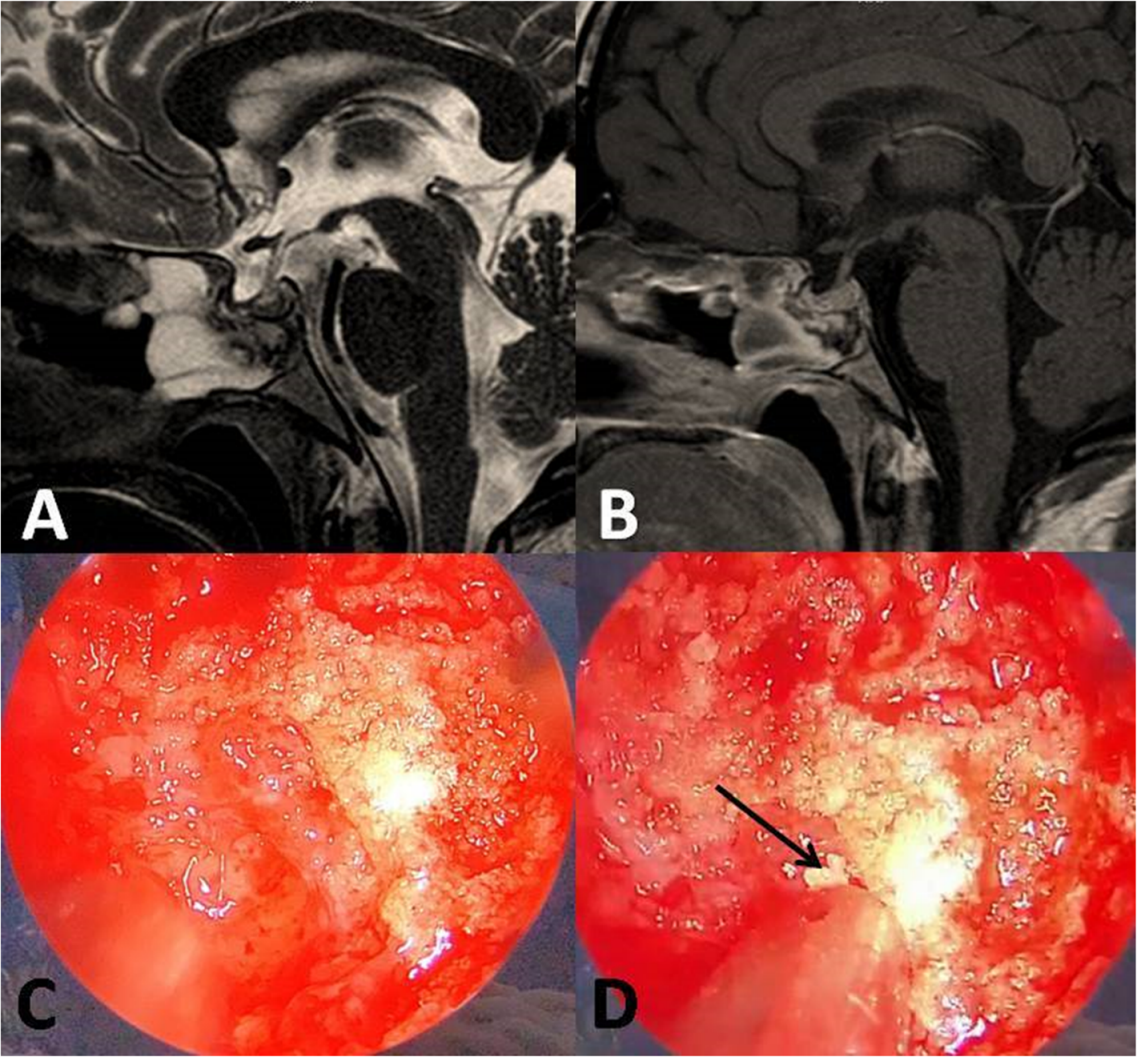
a) T2-weigthed and b) post-contrast sagittal brain MRI showing inflammation and thickening of the spheniodal and para-sphenoidal mucosa. c and d) Intraoperative endoscopic view showing the solid glass-like mass (acrylic glue) was seen in the inferior and lateral areas to the sphenoid sinus surrounded with inflamed infected mucosa. De-crustation of the mass shows fragmentation of the mass with glass-like particles *(arrow)*
